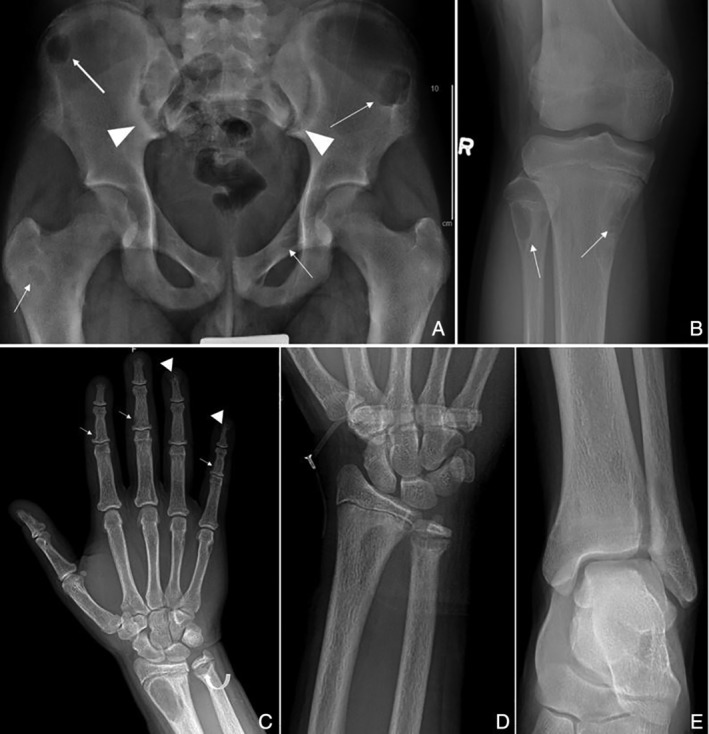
Anteroposterior (AP) radiograph of the pelvis (*A*) and right knee (*B*): Multiple well‐defined, lytic lesions (arrows) seen throughout the pelvis and proximal tibia and fibula with thin sclerotic borders compatible with brown tumors. Subchondral resorption at the sacroiliac joints with widening and adjacent sclerosis is more pronounced on the iliac side (arrowheads). AP radiograph right hand (*C*): Subperiosteal resorption along the radial aspects of the middle phalanges (arrows), and resorption of the distal phalangeal tufts consistent with acro‐osteolysis (arrowheads). Subphyseal resorption along the distal ulnar metaphysis resulting in physeal widening (curved arrow). AP radiograph of the left wrist (*D*): Site of HRpQCT distal radius scan, and left ankle (*E*): Site of HRpQCT distal tibia scan.
